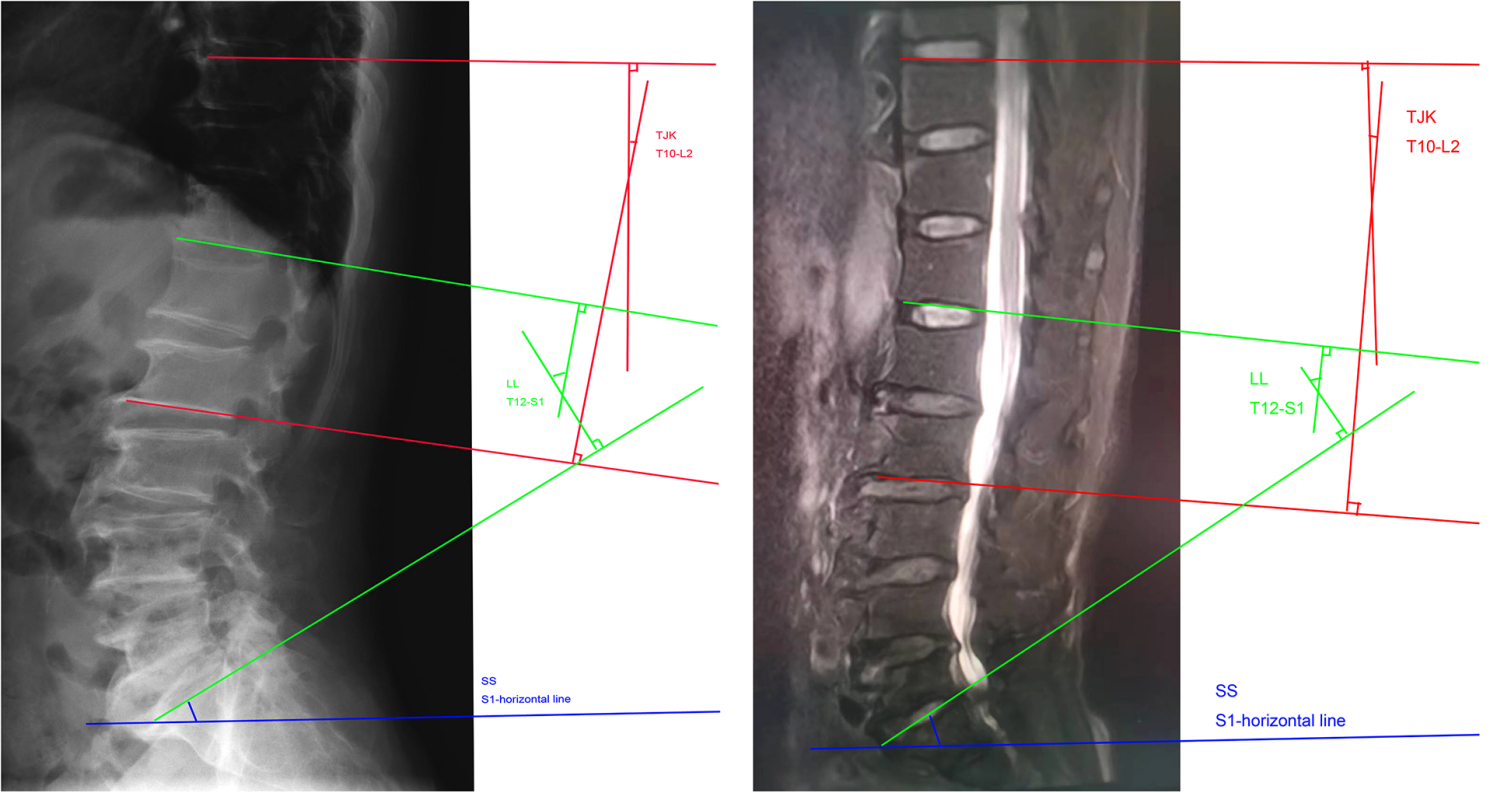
x-ray (side view) and MRI images with angle measurement marks. (A) X-ray imaging, (B): MRI imaging. MRI, magnetic resonance imaging.
